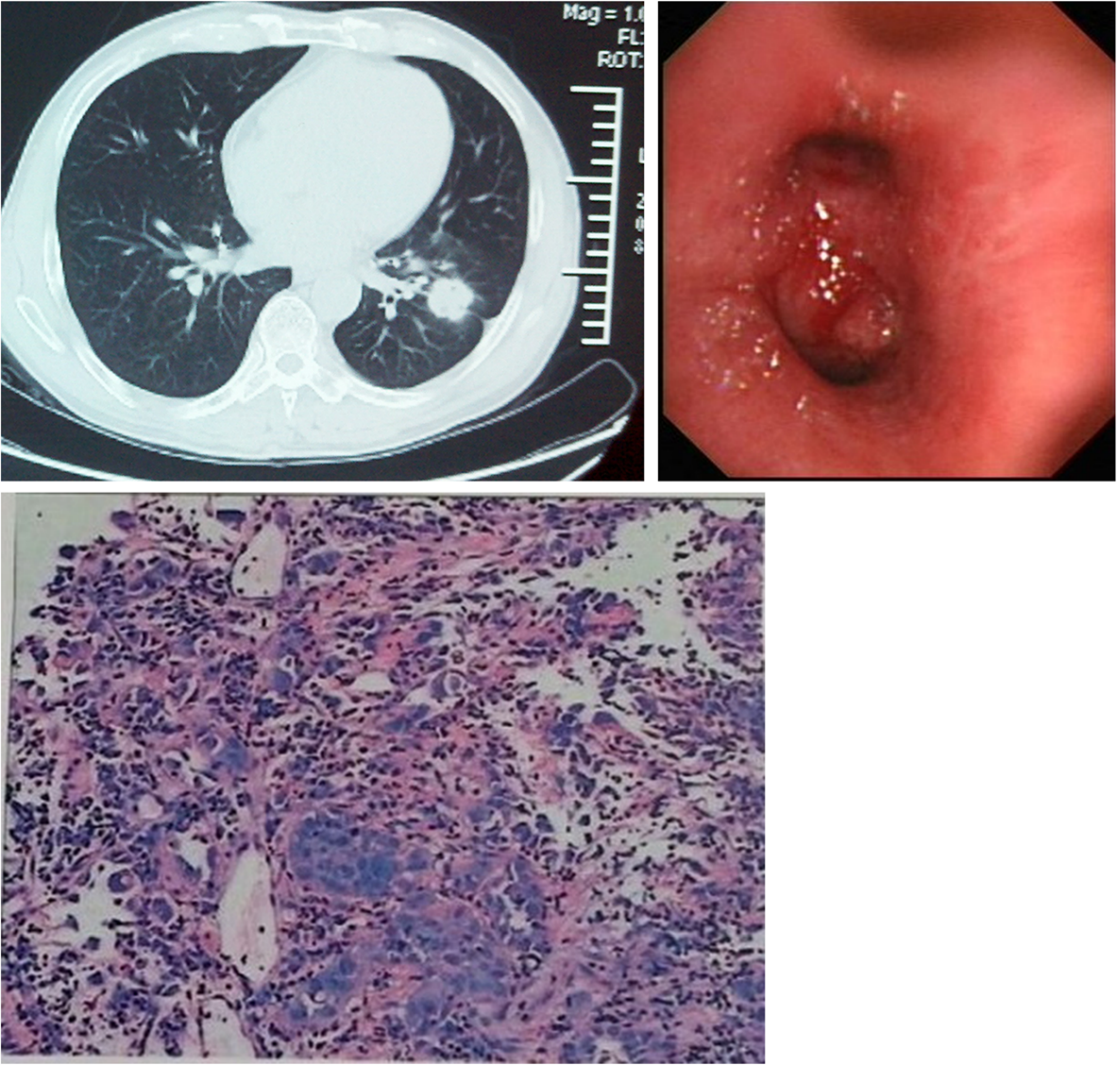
CT features and endobronchial metastasis. Endobronchial metastasis in peripheral lung adenocarcinoma. CT scan showing lung cancer in the medial anterior basal segment of left lower lobe, with pleural retraction. Appearance obtained by bronchoscopy. Left lung lower lobe and upper lobe bronchial mucosal hyperemia, infiltration change, and luminal stenosis. Endoscopic biopsy pathology, adenocarcinoma; EGFR mutation, exon 19 deletion mutation
